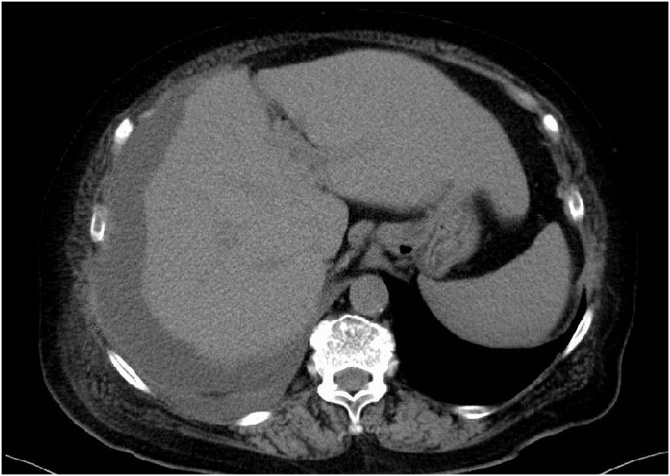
Image from abdominal enhanced computed tomography (CT) after the second PTGBA. CT revealed a large well-circumscribed fluid collection between abdominal wall, diaphragm and liver.
